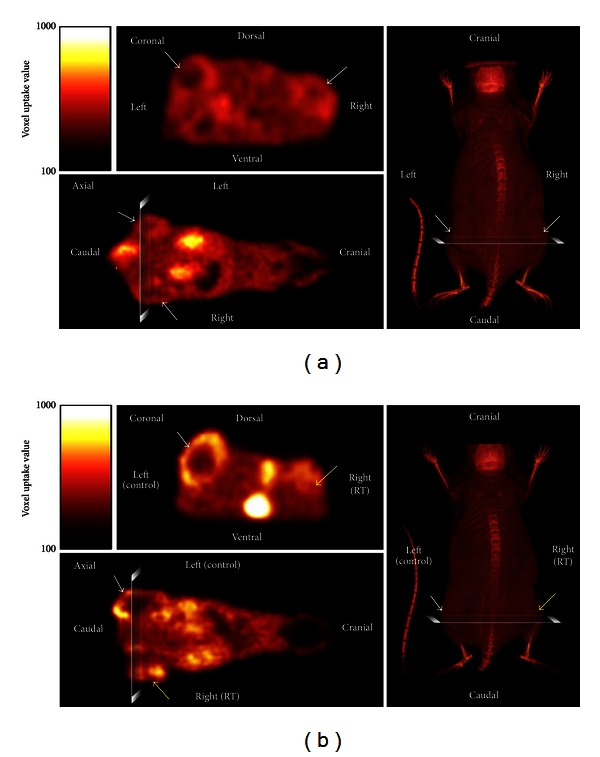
FLT-PET imaging demonstrates RT-induced changes in tumor cores. (a) ^18^F-FLT-PET of bilateral flank tumors prior to unilateral 16 Gy RT. Note the similar pattern of radiotracer uptake bilaterally in coronal and axial views (white arrows). 3D CT/bone imaging for flank surface anatomy is shown. (b) ^18^F-FLT-PET of bilateral flank tumors 2 weeks after unilateral 16 Gy RT. Unique variations in radiotracer uptake are seen. Note decreased radiotracer in control tumor core (white arrows); uniform uptake in RT tumors (yellow arrows). 3D CT/bone imaging for flank surface anatomy is shown.
